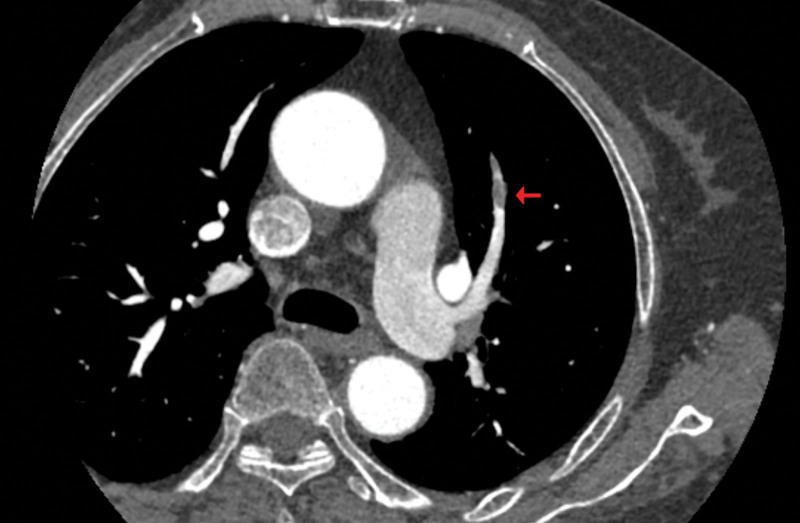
CT pulmonary angiography. Pulmonary thromboembolism in a branch of the left pulmonary artery (red arrow). CT = computed tomography.
